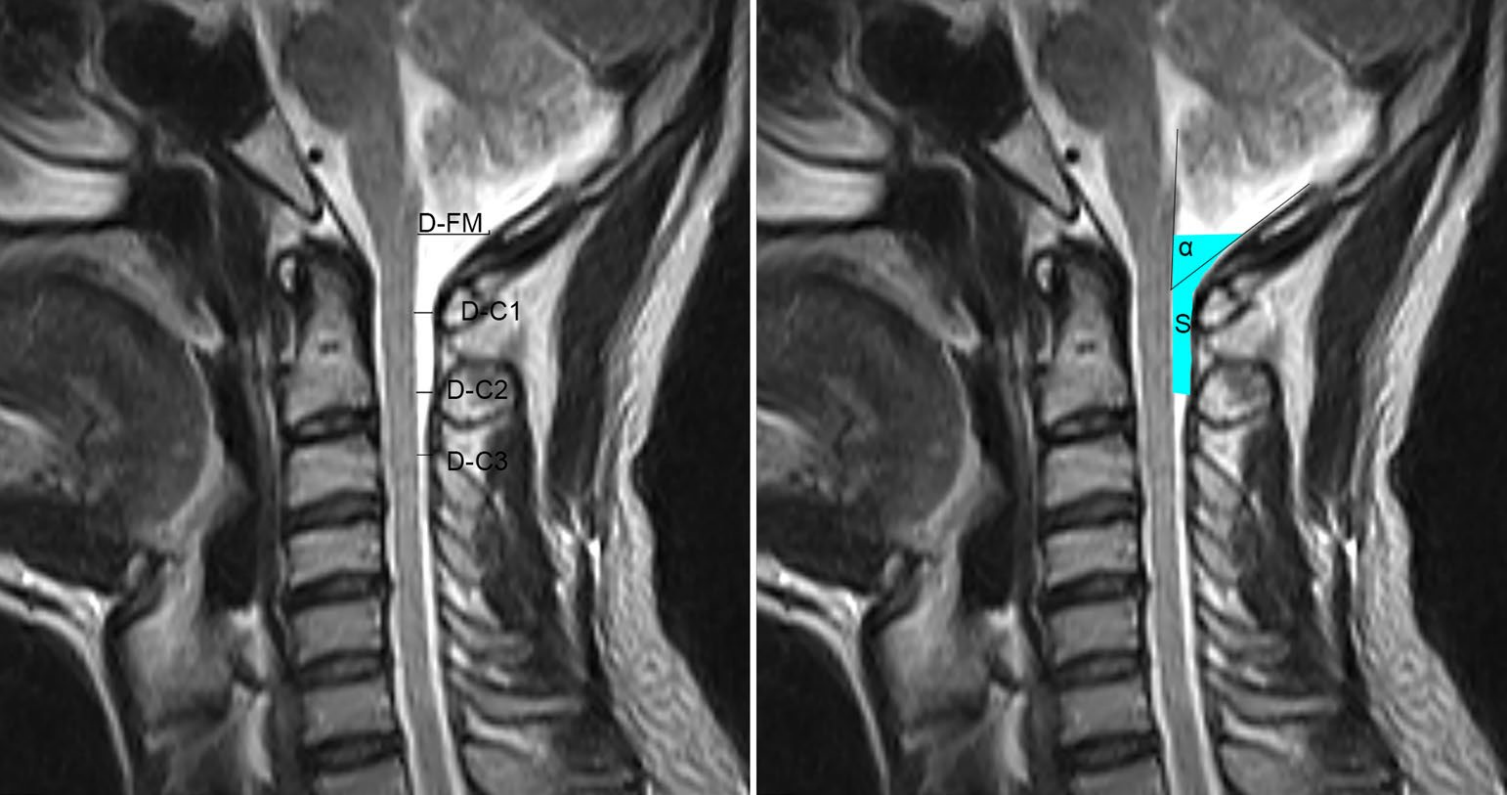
Median sagittal MRI of the head and neck showing the measurement parameters used to describe the enlarged subarachnoid space. D-FM, the anteroposterior extension of the subarachnoid space at the level of the foramen magnum (C0), measured horizontally from the posterior rim of foramen magnum (C0) to the dorsum of the medulla oblongata; D-C1, the anteroposterior extension of the subarachnoid space at the level of the atlas (C1), measured horizontally from the anterior point of the inner-convex surface of the arch of the atlas (C1) to the dorsum of the spinal cord; D-C2, the anteroposterior extension of the subarachnoid space at the level of the axis (C2), measured horizontally from the anterior point of the inner-convex surface of the arch of the axis (C2) to the dorsum of the spinal cord; D-C3, the anteroposterior extension of the subarachnoid space at the level of the third cervical vertebra (C3), measured horizontally from the anterior point of the inner-convex surface of the arch of the third cervical vertebra (C3) to the dorsum of the spinal cord; The spino-dural angle (SDA, α) of the enlarged subarachnoid space, which is formed by the dorsal side of the spinal cord and the dura extending a line passing through the posterior rim of the foramen magnum (C0) and the inner surface of the posterior arch of the atlas (C2); The median sagittal area (MSA, S) of the enlarged subarachnoid space dorsal to the spinal cord, calculated from the level of the foramen magnum (C0) to the level of the second cervical vertebra (C2) on the sagittal plane.
